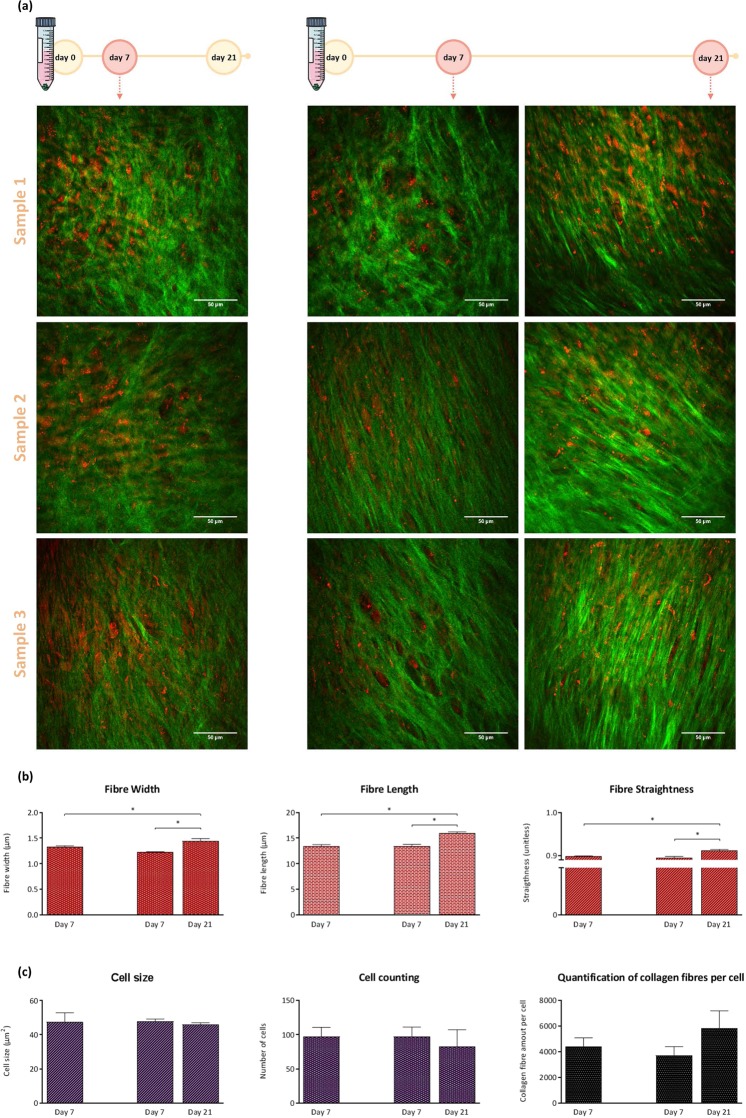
(a) Label-free live-imaging at day 7 and day 21 of fetal (human) femur-derived skeletal cell pellets cultured in chondrogenic media for 21 days. SHG imaged fibres of collagen (green) and lipid droplets (red) within the pellet of the neo-cartilage were imaged by CARS. Scale bars correspond to 50 µm. (b) Quantification of the width, length and straightness of collagen fibres. CT-FIRE was used to extract these details from the SHG images of collagen fibres. (c) Fiji was used to analyse CARS images to quantity the number of cells and their sizes for each cartilage pellet. Quantification was carried out to evaluate the amount of collagen fibres found per number of cells in the neo-cartilage tissue using both CARS and SHG data. Average data from 3 independent samples is shown where error bars indicate the observed standard deviation. The Mann-Whitney test was used to calculate the level of significance at p*-value < 0.05.
